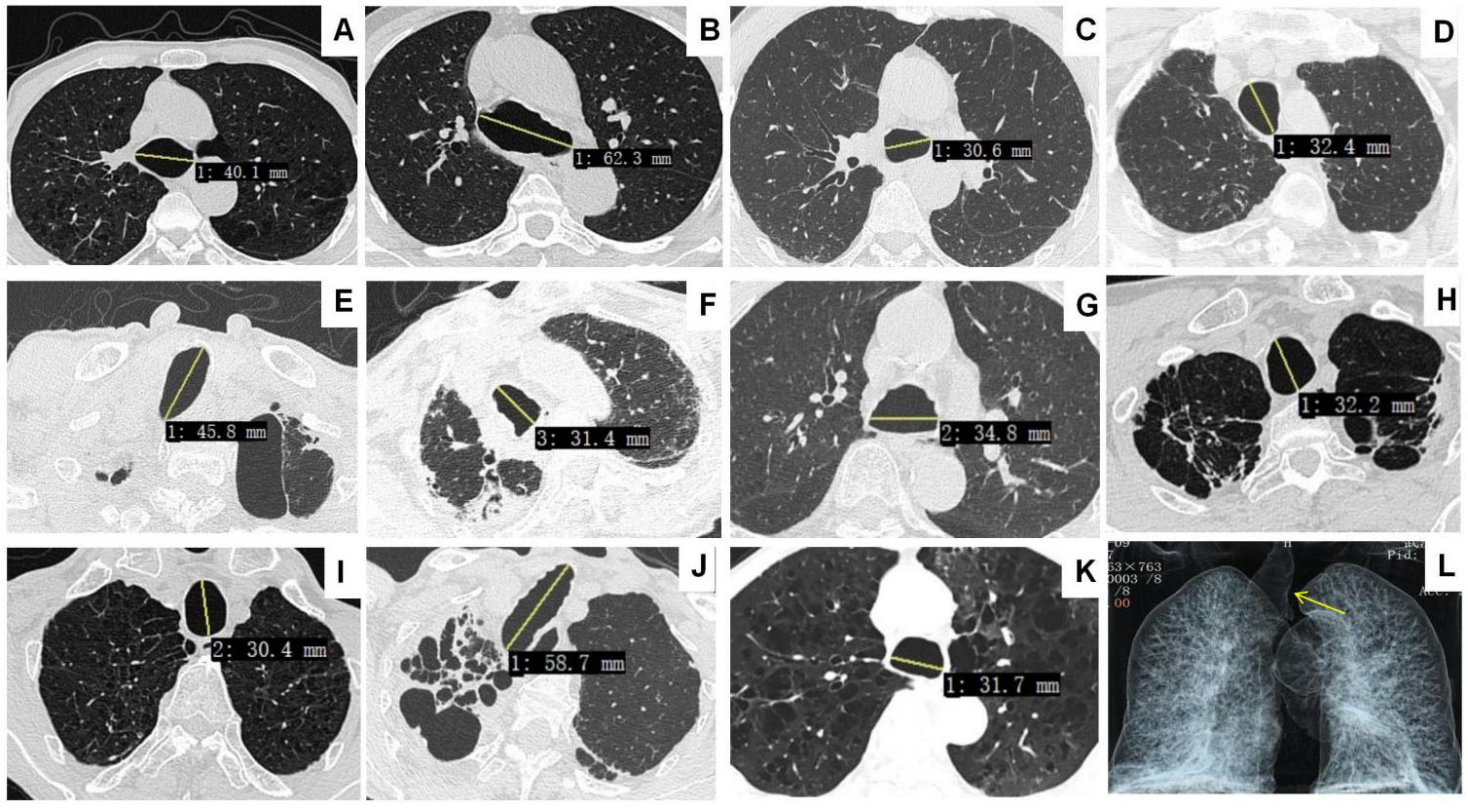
Chest CT showing tracheomegaly in Cases 1–11 (A–K). Airway reconstruction reveals main bronchial dilatation in case 2 (L, yellow arrow).
